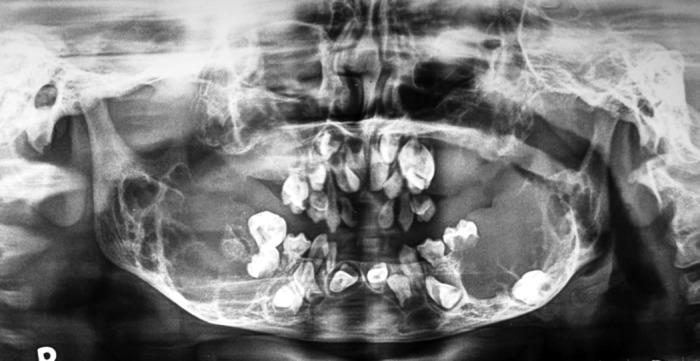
Radiograph showing multiple bilateral radiolucent
